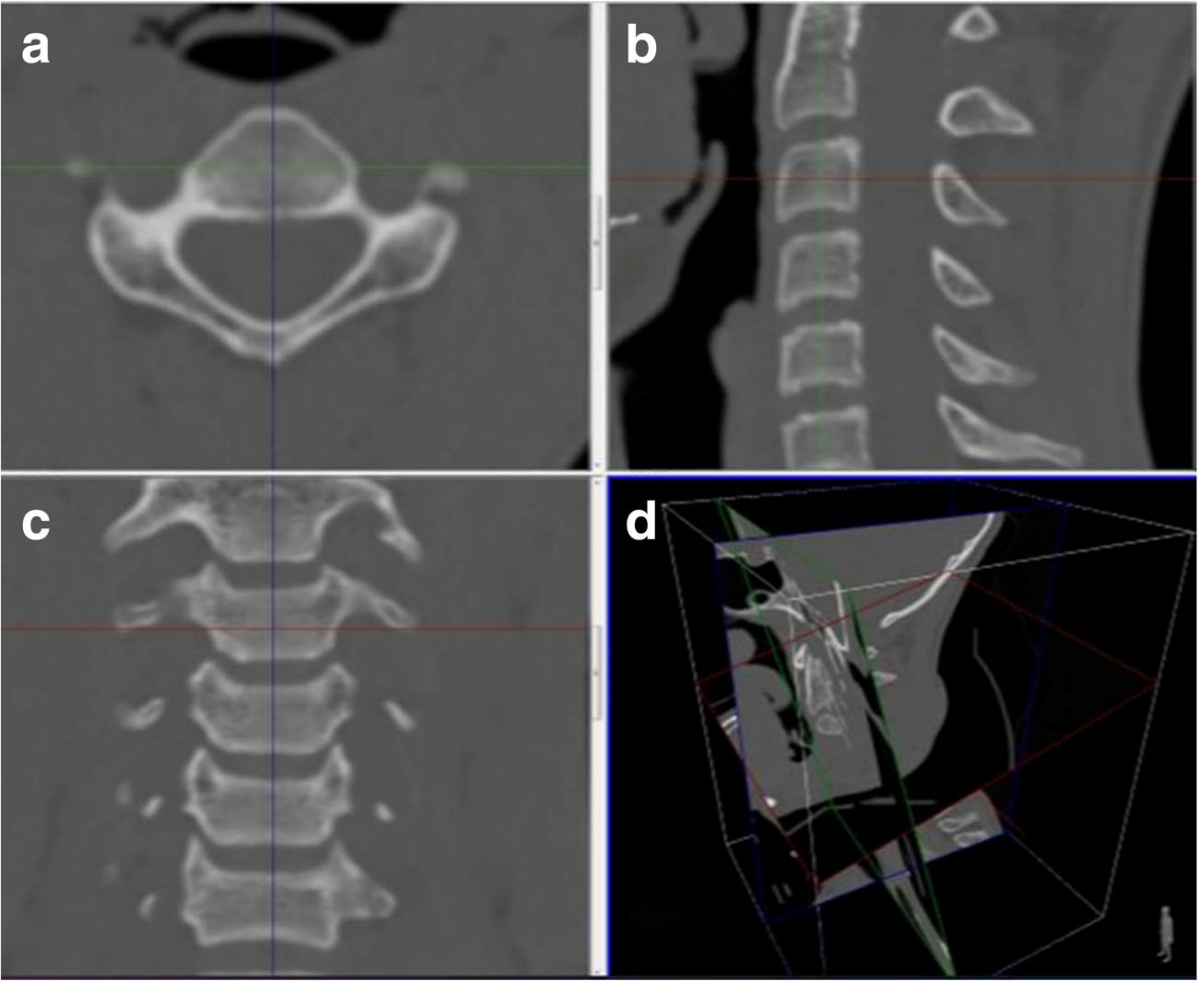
Determination of the CHT with axial (a), sagittal (b), coronal (c), and three-dimensional projections of section plans (d) reconstructed CT images
